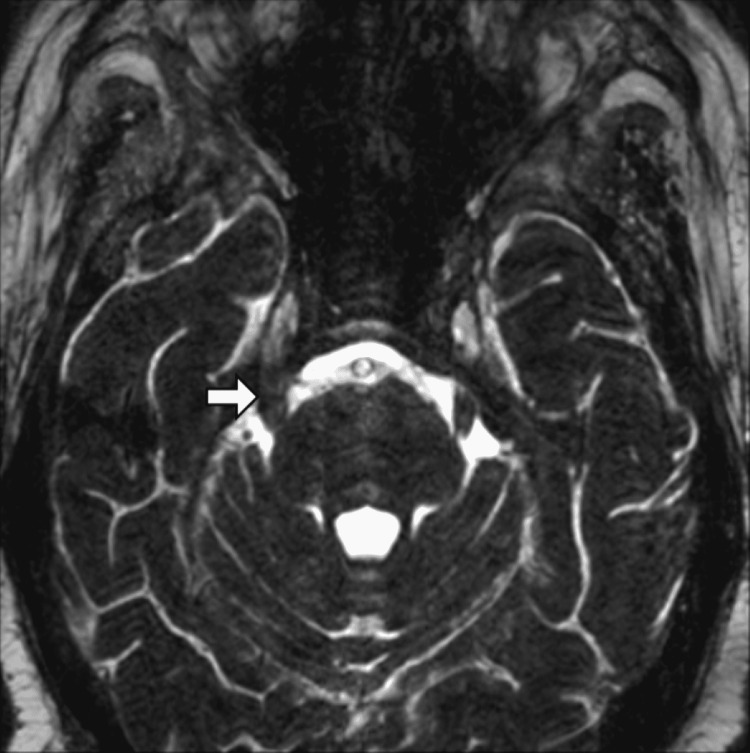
MRI of the brain in axial cut showing enlarged right trigeminal nerve (white arrow). MRI: Magnetic resonance imaging
